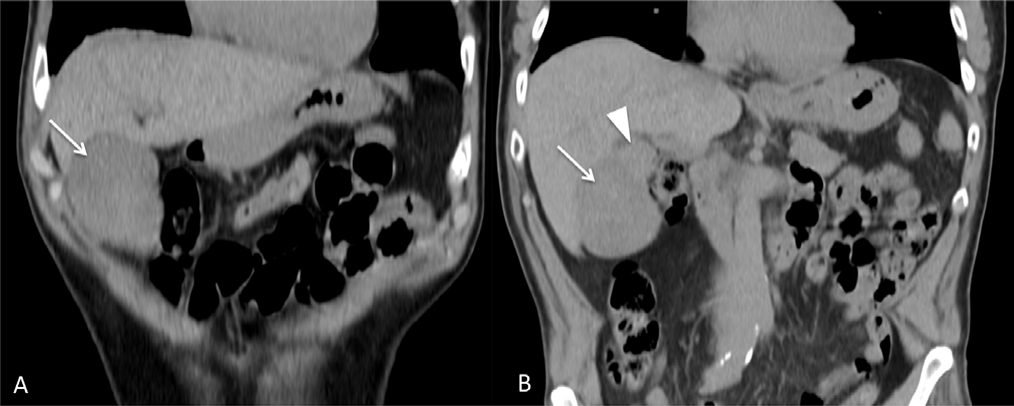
Coronal MPR precontrast CT images show a huge hypodense lesion along liver
